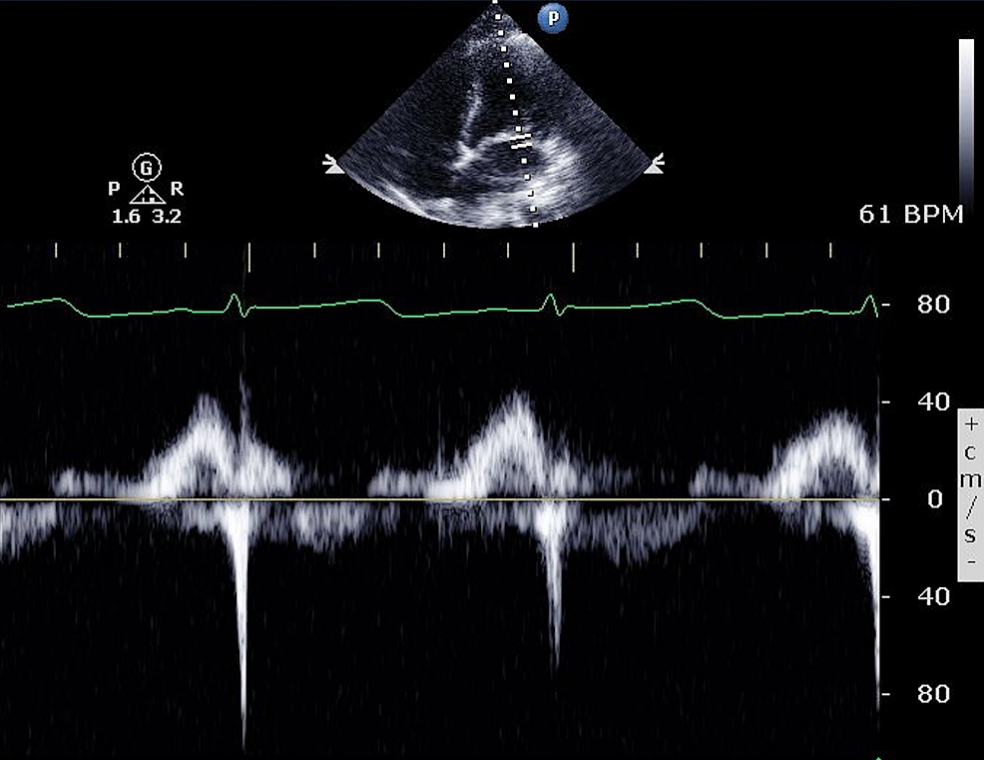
Initial echocardiogram showing pulsed-wave Doppler thought the mitral valve
